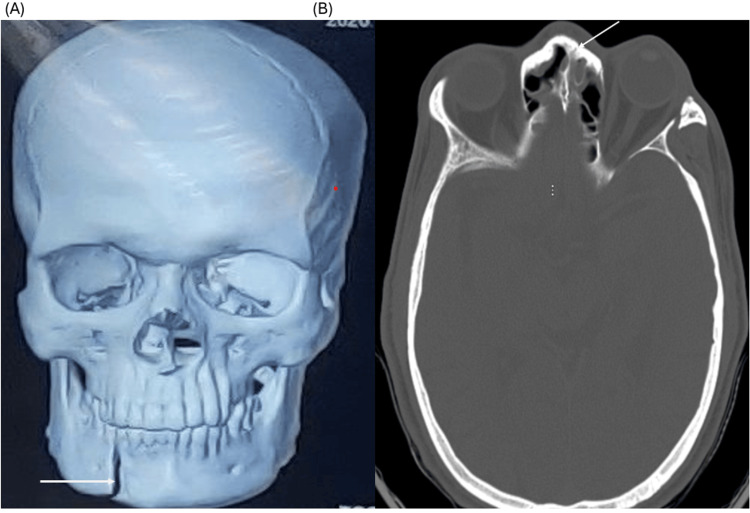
Preoperative CT scan showing facial fractures (A) Three-dimensional reconstruction demonstrating parasymphysis fracture of the mandible (arrow). (B) Axial CT section showing nasal bone fracture (arrow).
